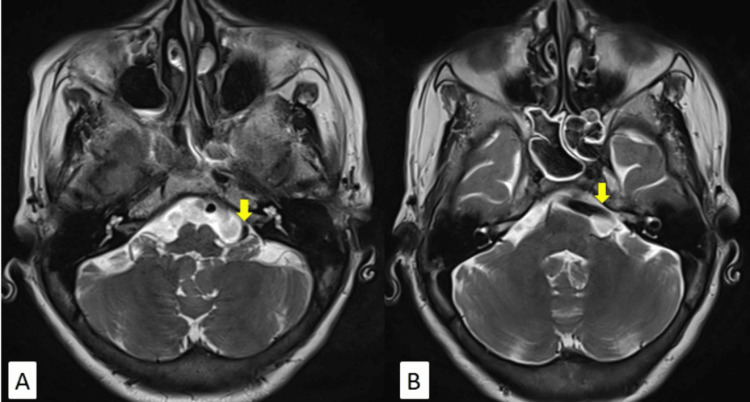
(A, B) MRI of the brain axial T2WI showing dolichoectasia of the left vertebral artery (yellow arrows) compressing the left VII-VIII nerve complex in the left CP angle cistern. T2WI: T2-weighted imaging; CP: cerebellopontine; MRI: magnetic resonance imaging
